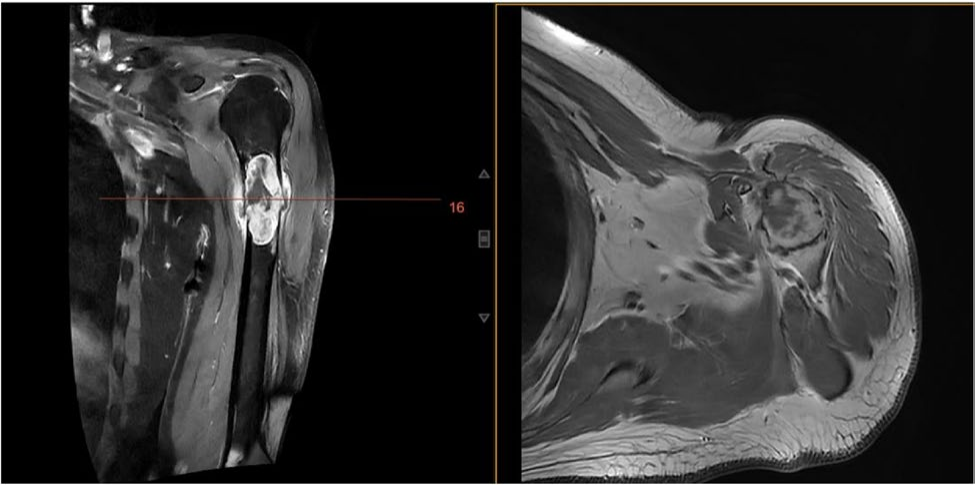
(MRI)The coronal(L) and axial(R) views show a mass-like abnormal enhancement signal within the upper medullary cavity of the left humerus, with the long axis of the lesion parallel to the long axis of the humerus
